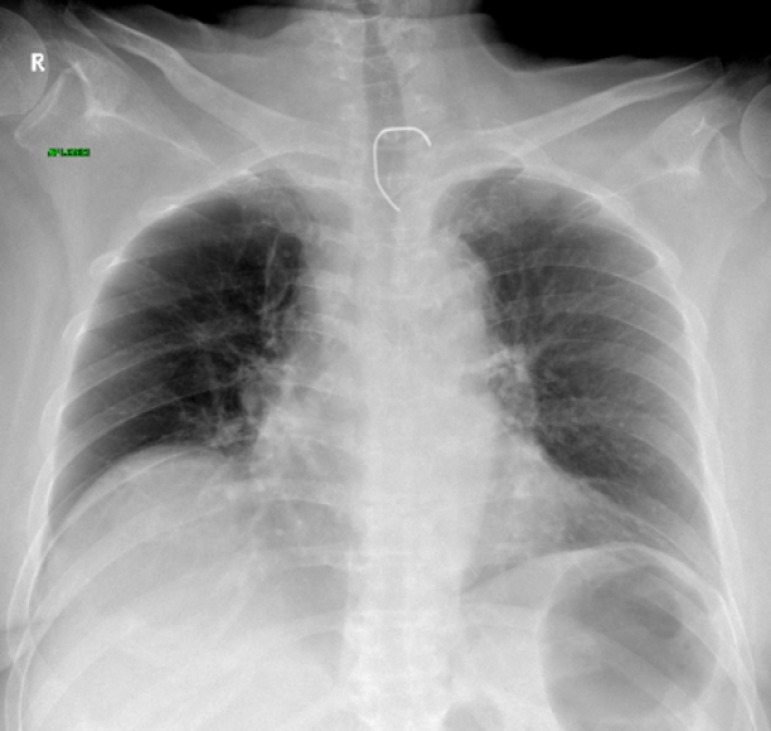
Chest X-ray showing foreign body.
